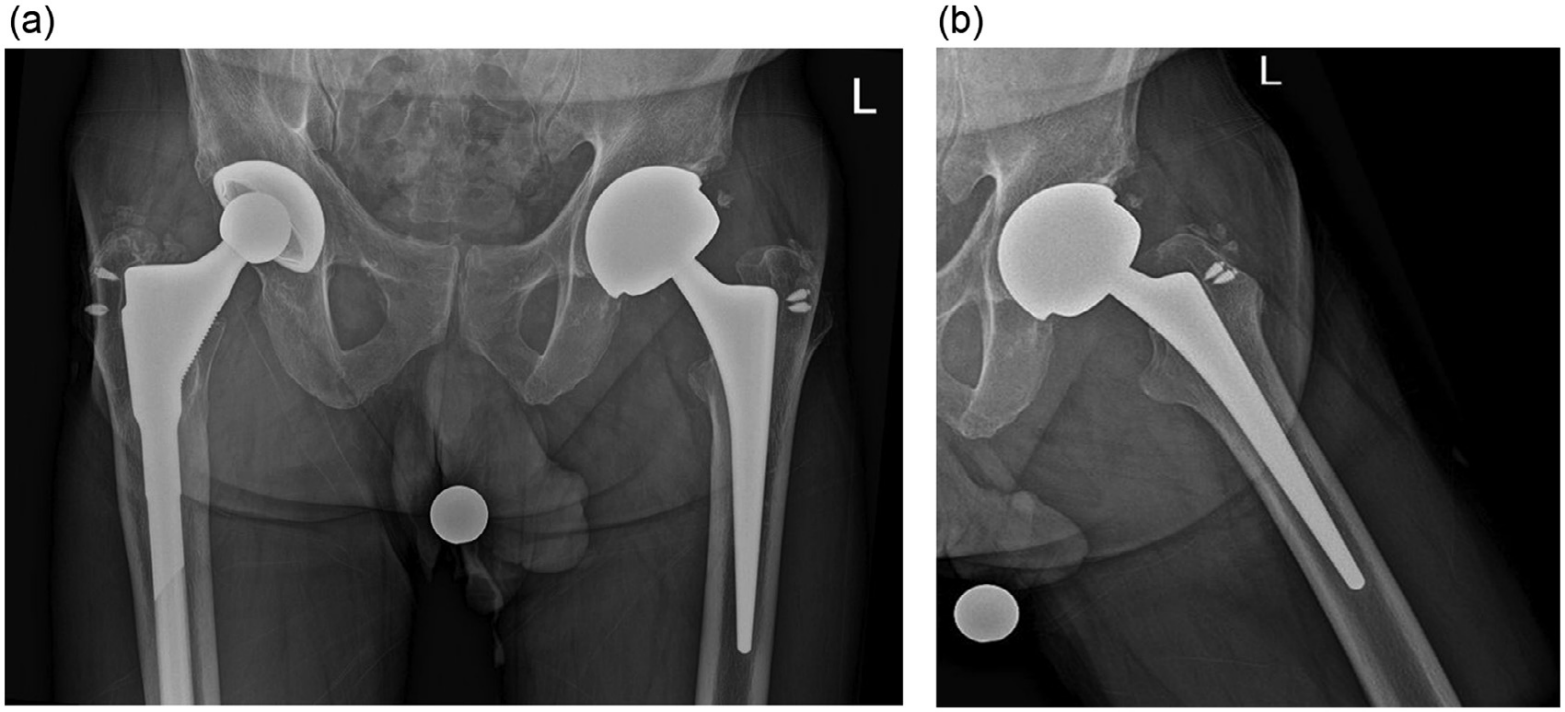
(a) Low-centered AP radiograph of the pelvis and (b) lateral radiograph of the left hip demonstrate a metal-on-metal left total hip arthroplasty. There is also a right total hip arthroplasty with a metal-on-polyethylene design. Suture anchors are present in the bilateral greater trochanters. No metallic debris is visible radiographically.
